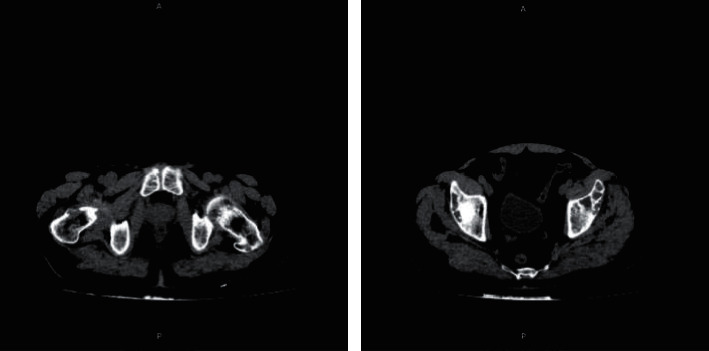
Contrast abdomen CT scans.
